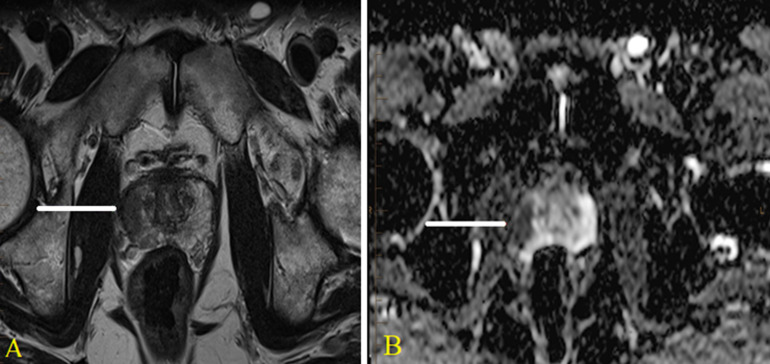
magnetic resonance imaging of the prostate showing - A) T2-weighted indicating lesion in the peripheral zone (white line); B) apparent diffusion coefficient (ADC) indicating focal mild to moderate hypointensity in the peripheral zone (white line)
